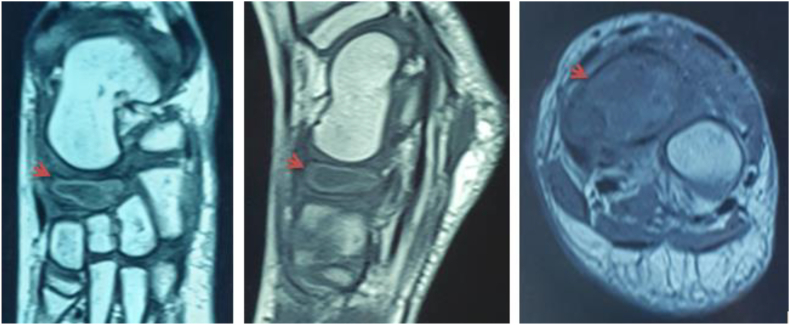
Unenhanced T1-weighted MRI sequences: T1 hypointense of the spongy bone of the navicular bone (arrows) on the coronal, sagittal and axial slices showing the “penumbra sign” described as perilesional lining of higher signal intensity, with a central hypo intense content.
